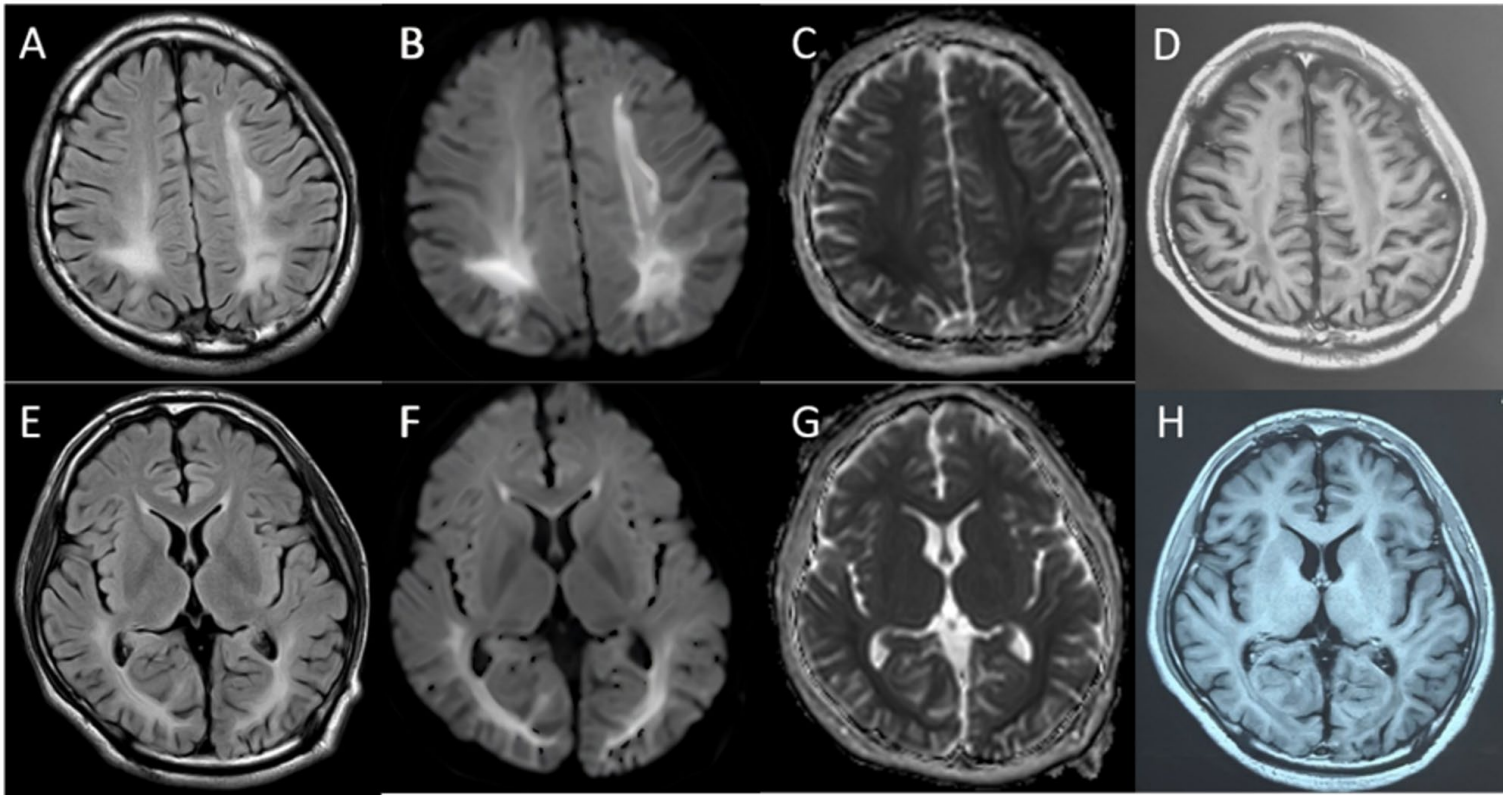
Axial FLAIR (A, E) MRI shows increased signal intensity in the frontal, parietal and occipital white matter. Matching axial plane DWI (B, F) and ADC (C, G) maps show restricted diffusion in the corresponding areas. Contrast-enhanced (D, H) showed no significant enhancement
